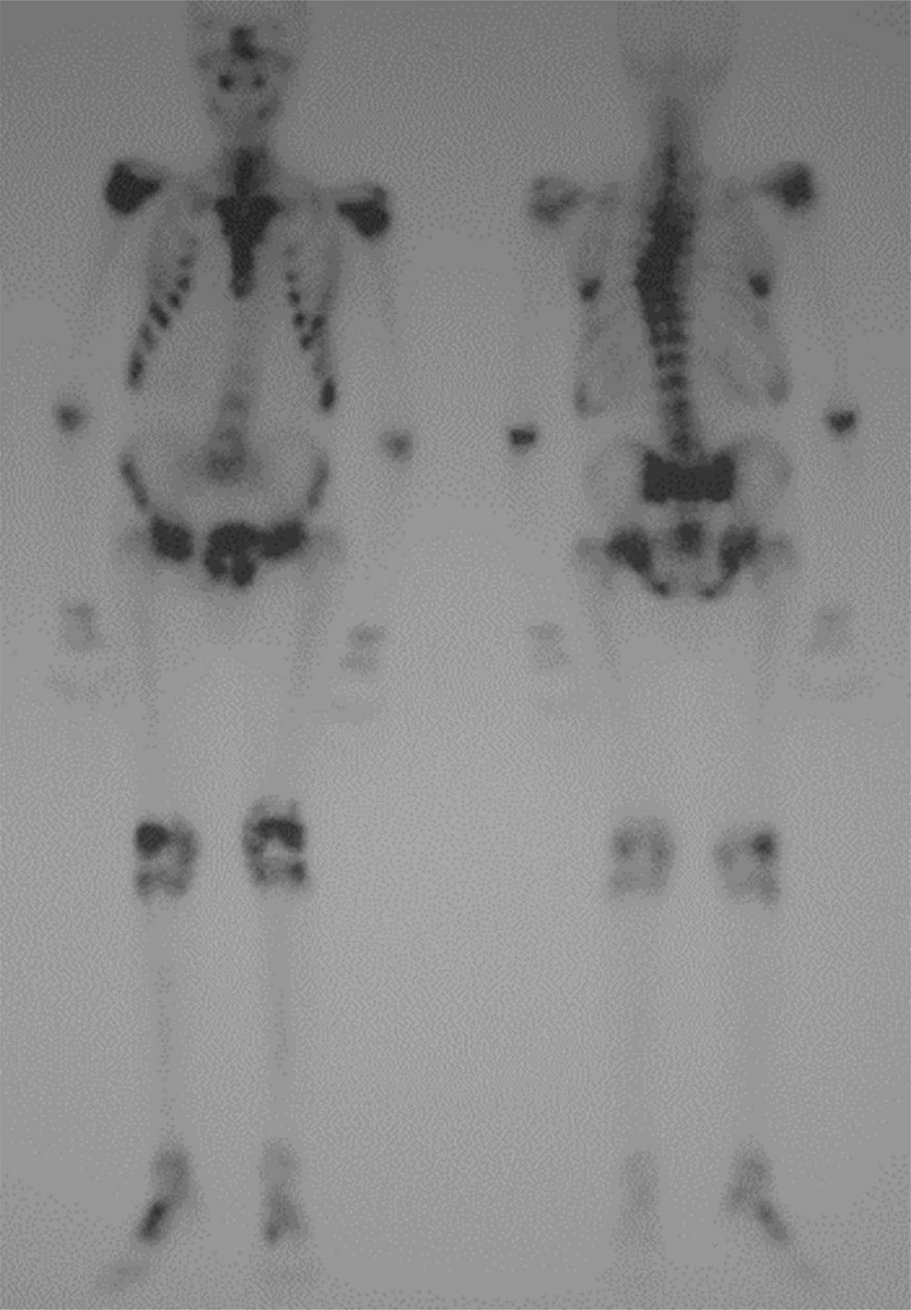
22-year-old man with a rhinopharyngeal vault PMT. Whole-body bone scintigraphy demonstrated multiple areas of increased uptake in the thoracic spine, ribs, pelvis, and limbs with a characteristic H-shape pattern in the sacrum, typically found in insufficiency fractures
